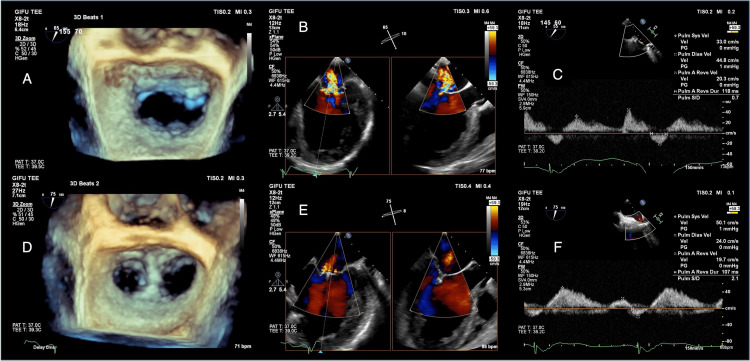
Perioperative transesophageal echocardiography A: pre-procedural three-dimensional mitral valve; B: pre-procedural two-dimensional mitral regurgitation; C: pre-procedural pulmonary vein flow; D: post-procedural three-dimensional mitral valve; E: post-procedural two-dimensional mitral regurgitation; F: post-procedural pulmonary vein flow
